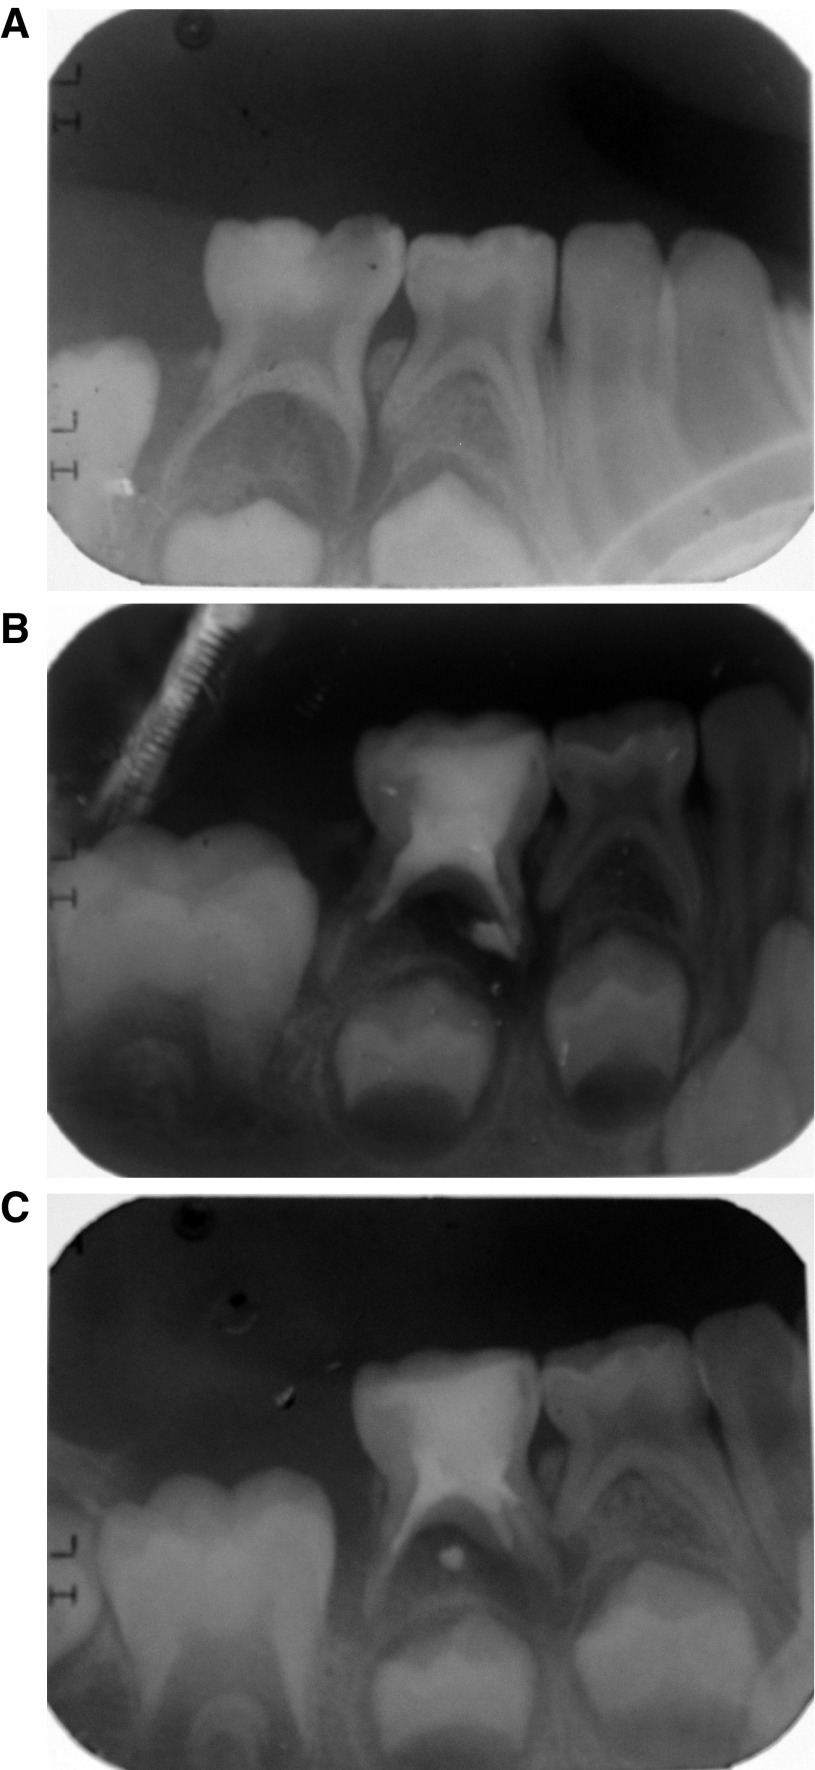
Radiographic examination. A) Pre-operative radiograph: Bone rarefaction and root resorption in first and second primary molars;
